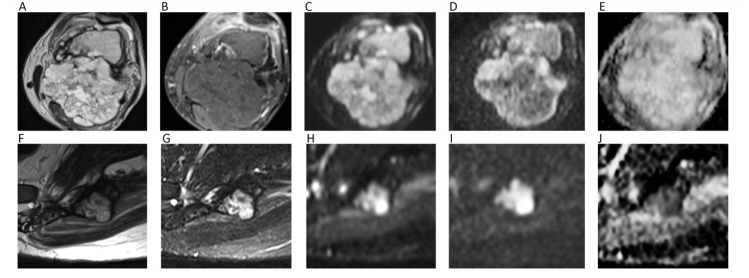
The left femur G3 chondrosarcoma (A–E) of a 76-year-old male patient. Axial T2w image (A) shows a hyperintense bone lesion with a large posterior soft tissue mass presenting poor contrast enhancement on fat-suppressed T1w image (B). The lesion shows high signal on *b* = 0 DWI image (C), decreased signal on *b* = 1000 DWI image (D), and unrestricted pattern of diffusion on the corresponding ADC map (E, mean ADC: 2.303 × 10^−3^ mm^2^/s). The Ewing sarcoma (F–J) of the left iliac bone of a 31-year-old male patient. The tumor presents as an esofitic bone lesion with intermediate signal on axial T2w image (F) and high signal on axial STIR image (G). The lesion shows intermediate-high signal on *b* = 0 DWI image (H), higher signal on *b* = 1000 DWI image (I), and restricted pattern of diffusion on the corresponding ADC map (J, mean ADC: 0.899 × 10^−3^ mm^2^/s).
